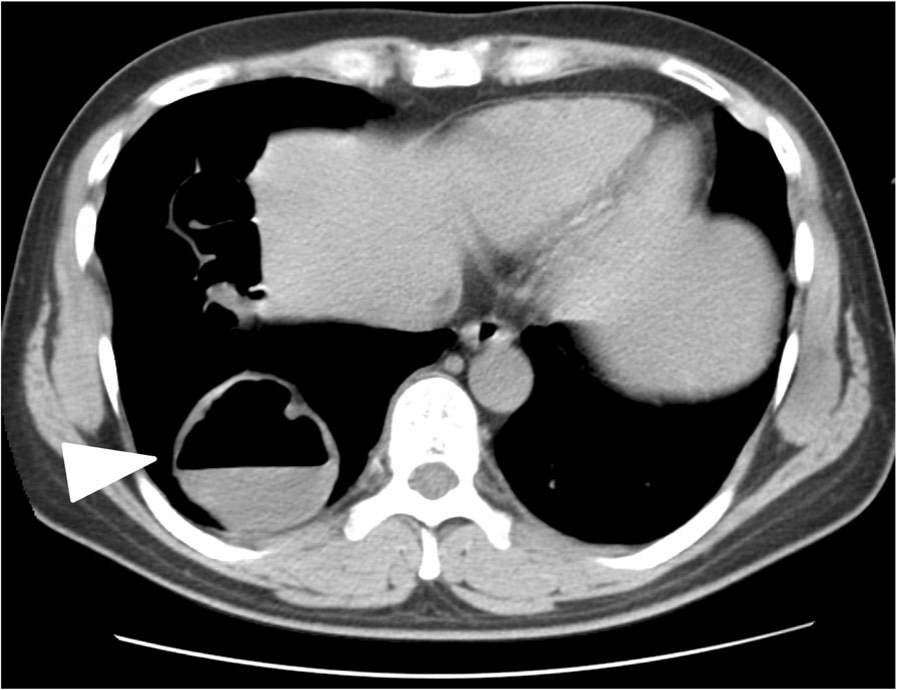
c Computed tomography showing the herniated transverse colon located in the right thoracic cavity (arrow head)
